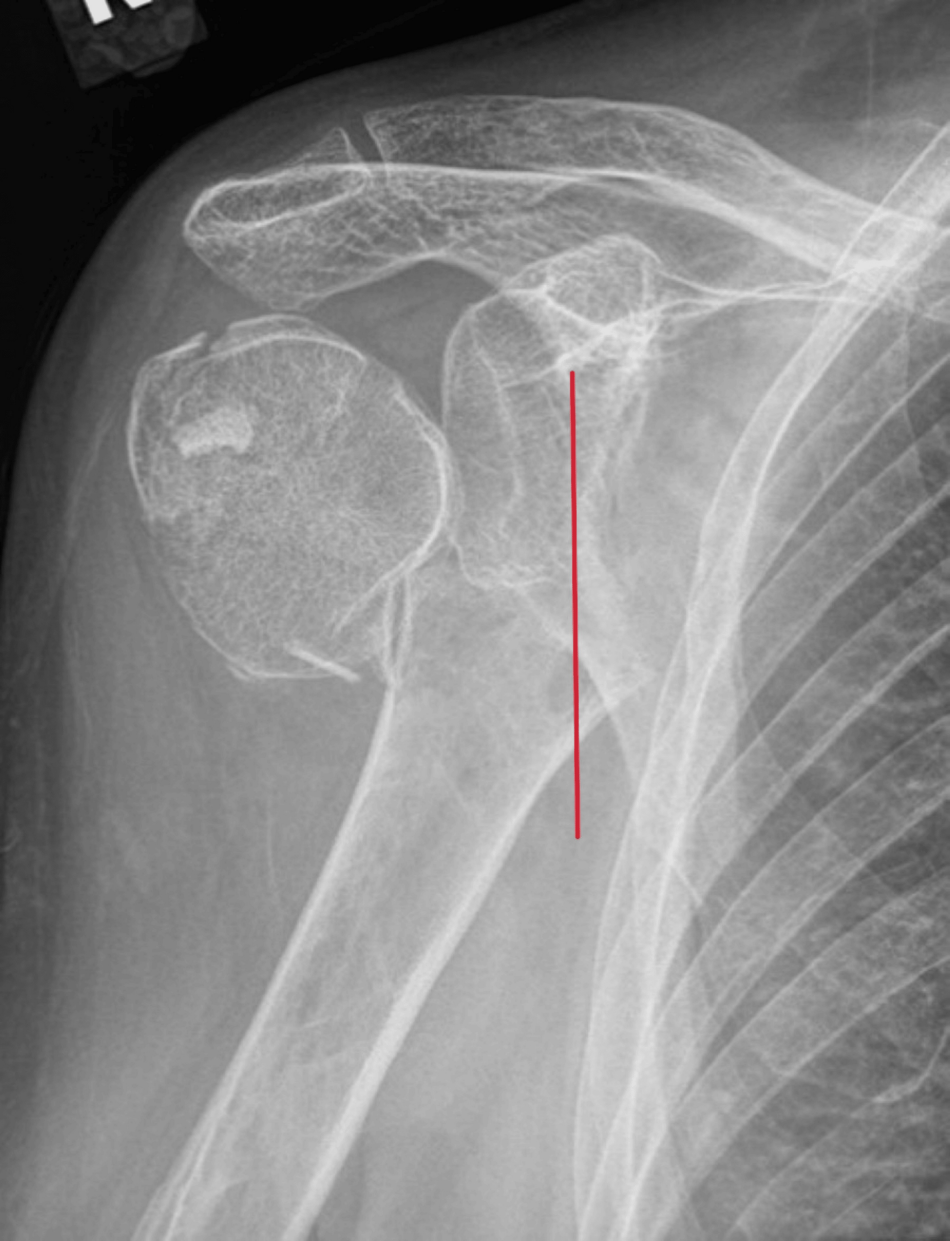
Preoperative anteroposterior radiograph of a 73-year-old female The line at risk shows significant medial displacement of the humeral shaft.
